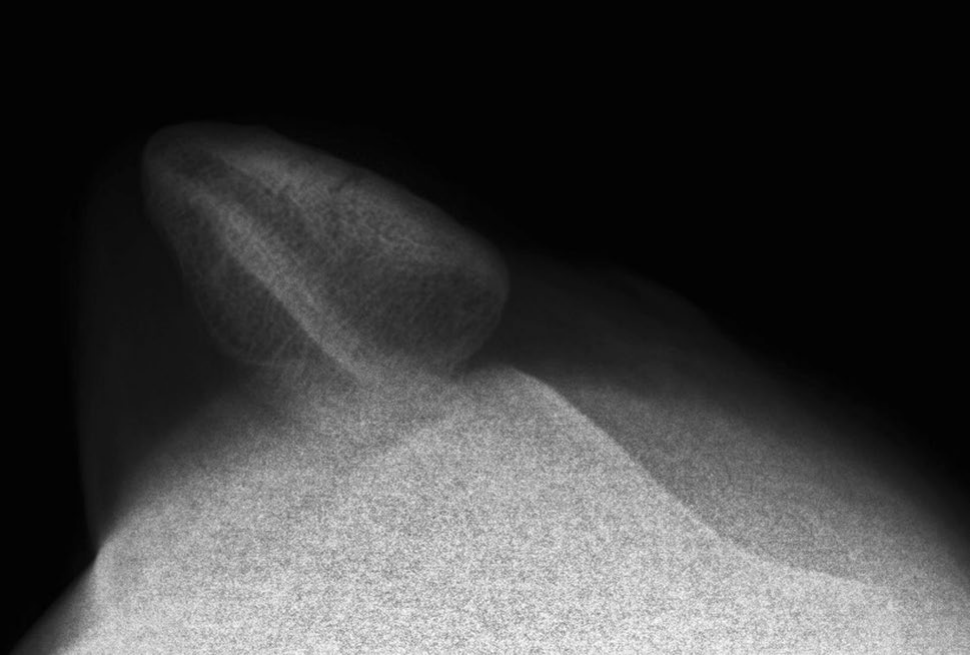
Right knee radiograph: axial view confirming the lateral dislocation without rotation in the longitudinal axis
